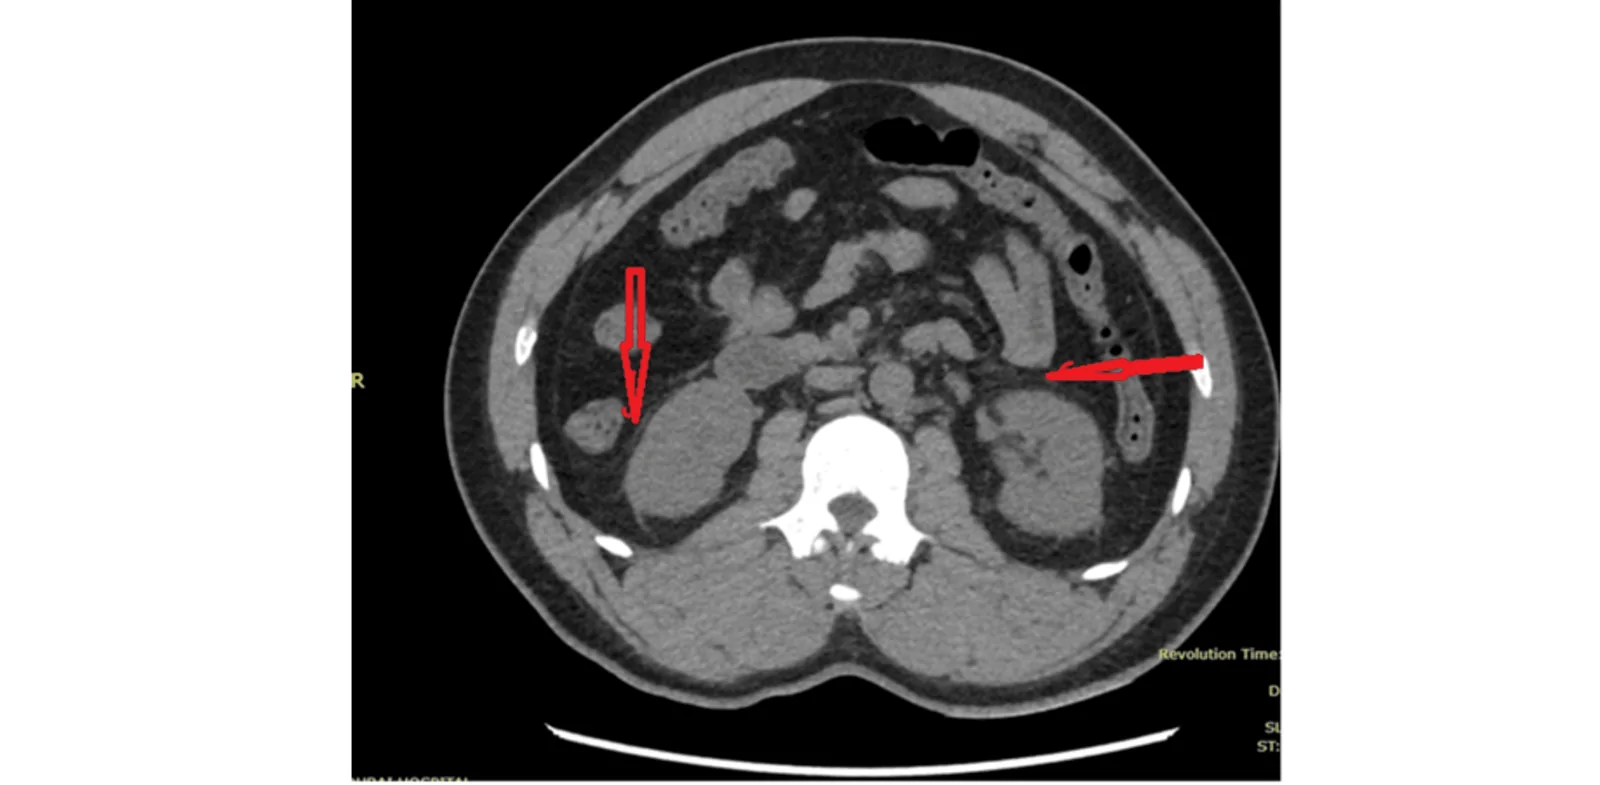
Contrast-enhanced CT of the abdomen revealed perinephric fat stranding and pararenal fascial thickening as pointed by arrows
